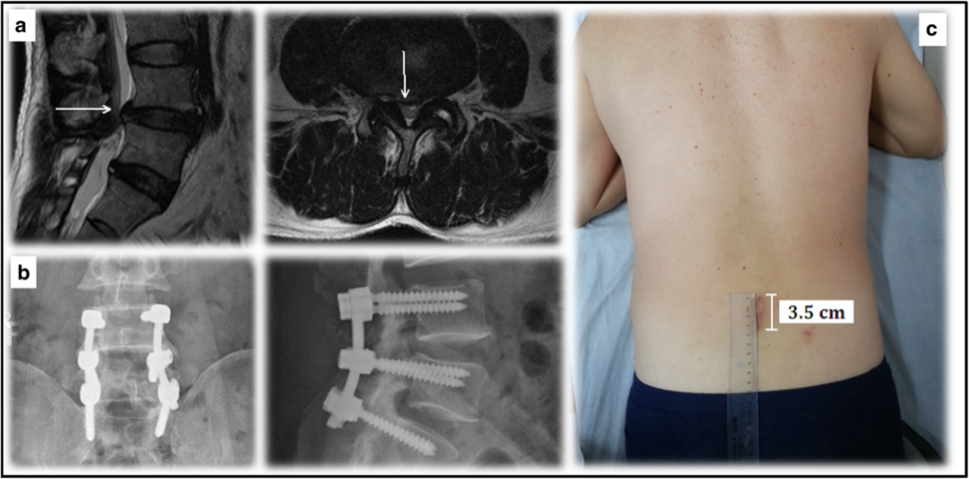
a MRI images showed disc herniation at L4/5 and L5/S1 levels (*arrows*). b A navigated PLF was performed. c The length of incision decreased from 4 to 3.5 cm in the 2 years after surgery
